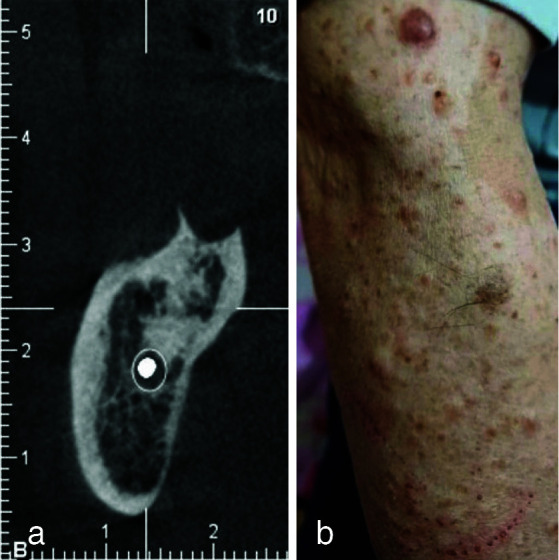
a: Cone-beam computed tomography (CBCT) (coronal view) of molar area showing a well-defined opacity. b: Skin examination showing multiple papules on the skin of hands
